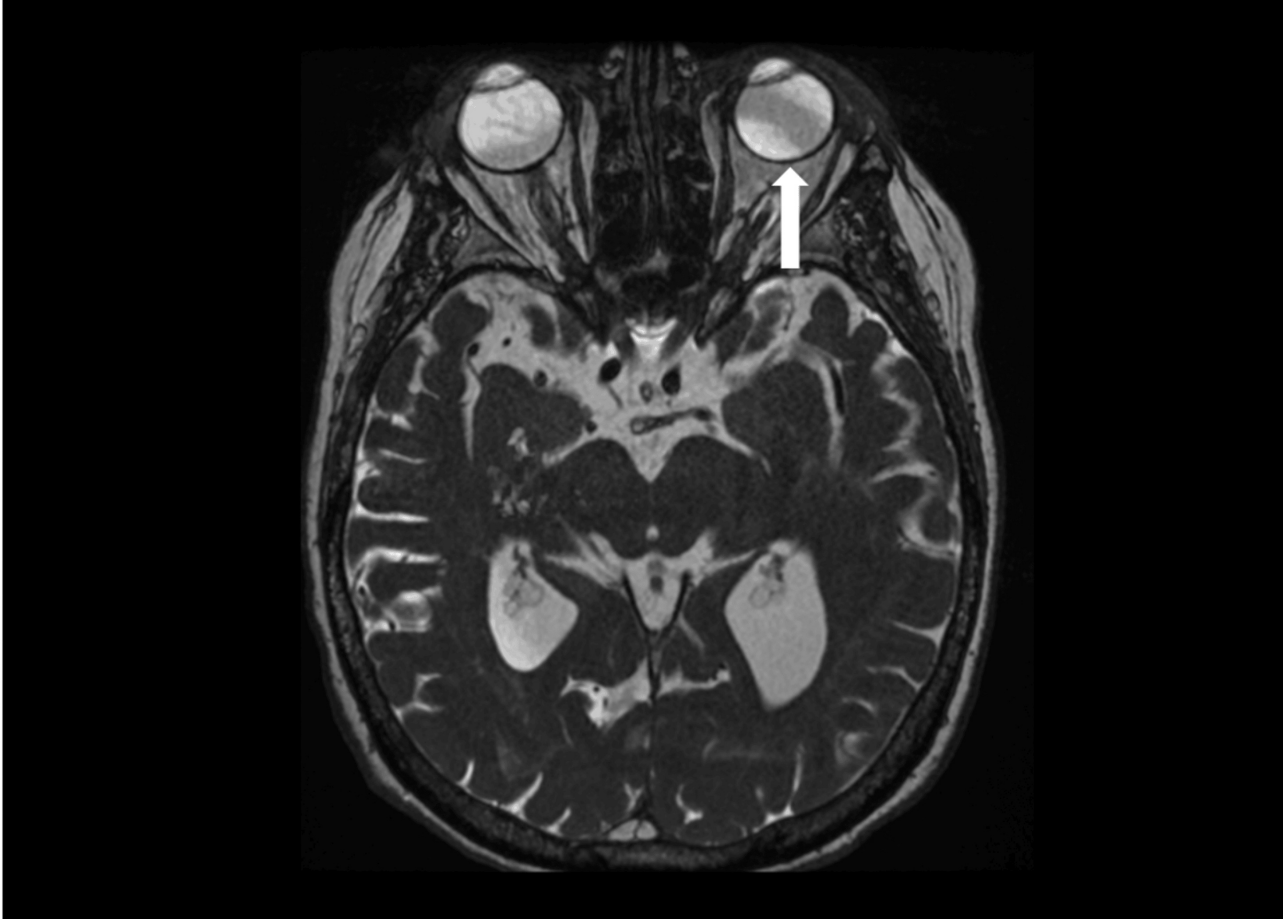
T2-weighted MR demonstrating left eye adduction due to vascular loop syndrome (arrow).
